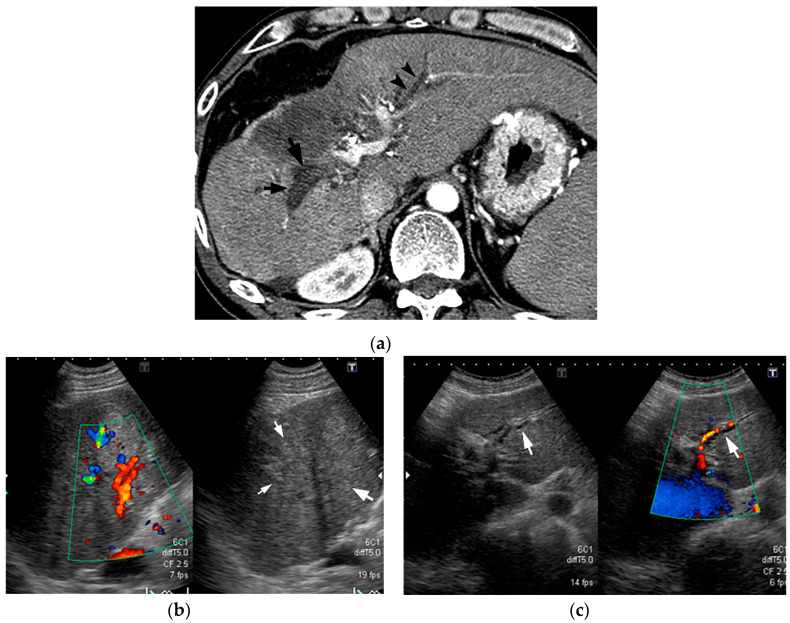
Comparison between CT and ultrasound (US) findings of BDN. (a) On contrast-enhanced CT, a hypodense thick tubular structure (arrow) is observed in the hepatic hilum of the right hepatic lobe, and in the left lateral segment, a similar hypodense thin tubular structure (arrowheads) is observed. Although these tubular hypodense structures look like dilated intrahepatic bile ducts, the right lobe lesion is BDN and the left lateral lobe lesion is bile duct dilatation. (b) On US images of the right hepatic lobe, no dilated bile ducts are observed in the portal tract. The portal tract shows iso- to slightly hyper-echo compared to the surrounding liver parenchyma (arrows). This US finding is inconsistent with the CT finding. (c) In the US image of left lateral lobe of the liver, a slightly dilated echo-free tubular structure (arrows) is observed along the arterial branch, which represents intrahepatic bile duct dilatation. As indicated on Figure 12b, BDN is iso- to slightly hyper-echo compared to the surrounding liver parenchyma and shows discrepancy with the CT finding.
